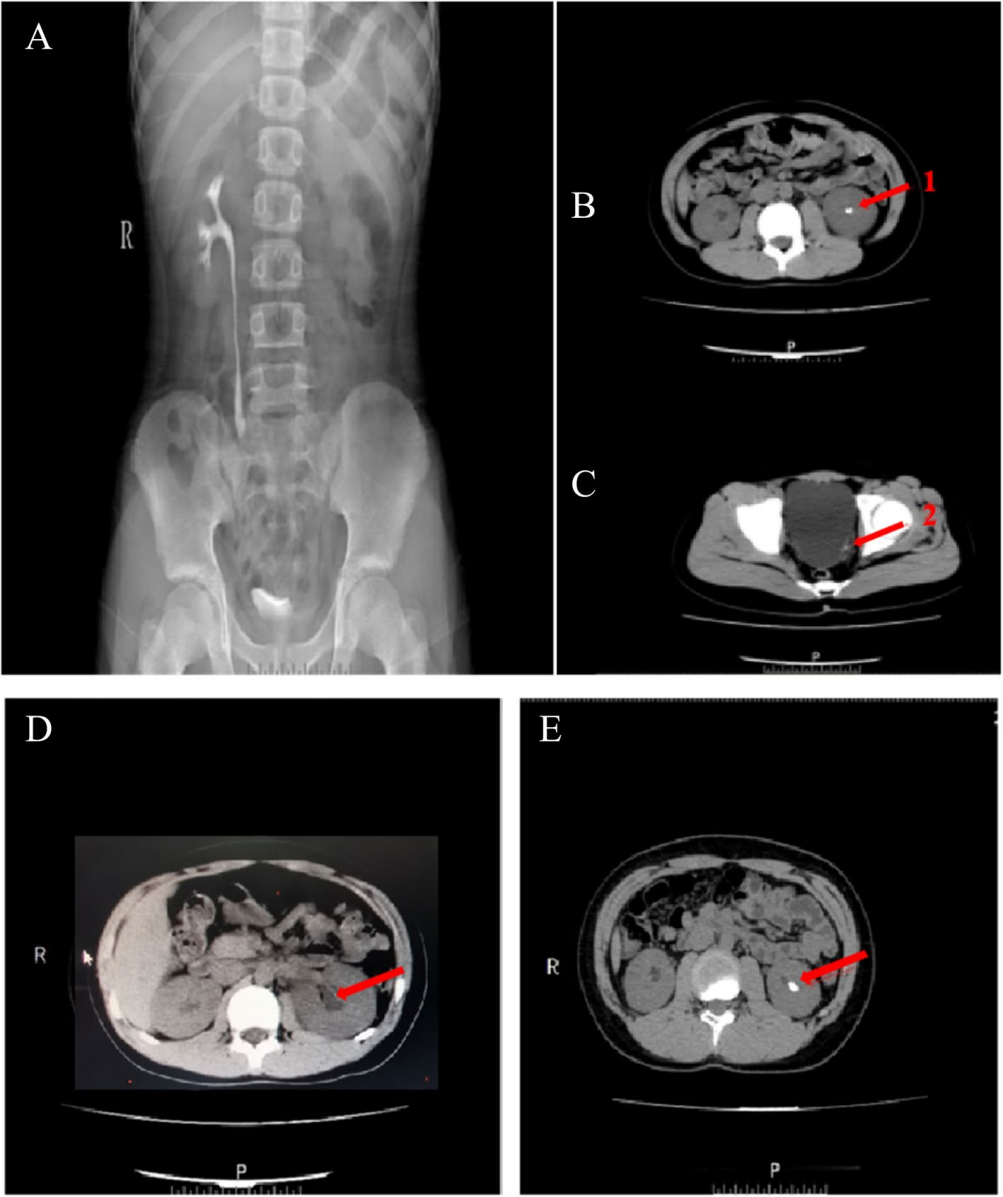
Radiographic findings in the patient. R: The right side of the body, P: The front of the body. A KUB film. B The red arrow indicates the stones in the left kidney (arrow 1); the average CT value of the arrow is 199.63 HU. C The red arrow indicates the stones in the left ureter (arrow 2). D Hydronephrosis was seen in the left kidney. E Six months later, residual fragments were found in his left kidney. The average CT value of the arrow is 752.25 HU. (The reference CT value for cystine stones is between 200 ~ 1100HU)
